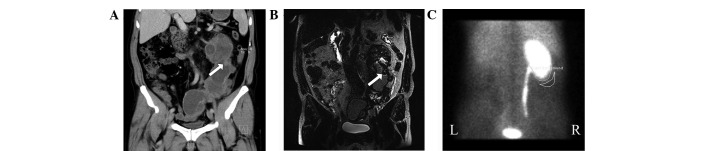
Imaging observations of patient 4. Coronal (A) contrast-enhanced computed tomography and (B) T2-weighted images showed the distention of the renal pelvis and ureter, as well as multiple nodular thickening of the wall of the ureter. (C) Fused image of the clearance phase of renal dynamic imaging failed to show the left kidney, which denoted that the renal function of the left kidney had been lost.
